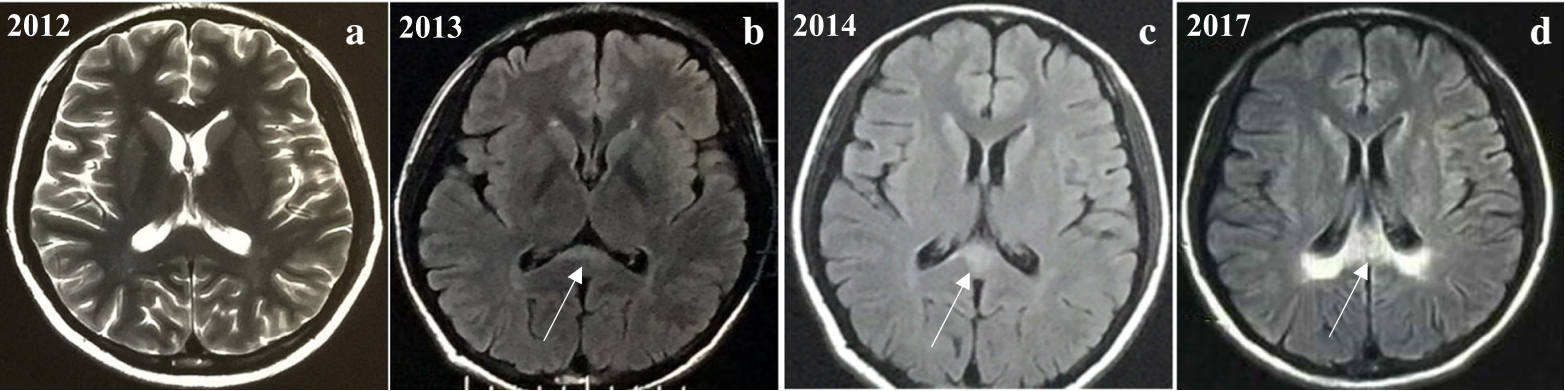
The evolution of brain MRI scans of patient 1 during last 5 years. a The axial T2-weighted images of brain MRI of 2012 is normal. As indicated by the arrows. b The axial T2-flair images of head MRI of 2013 showed a slight hyperintensity of splenium of corpus callosum. c The axial T2-flair images of head MRI of 2014 showed more abnormal signal of splenium of corpus than in 2013. d T2-flair images of head MRI of 2017 revealed marked hyperintensity of whole splenium of corpus callosum
